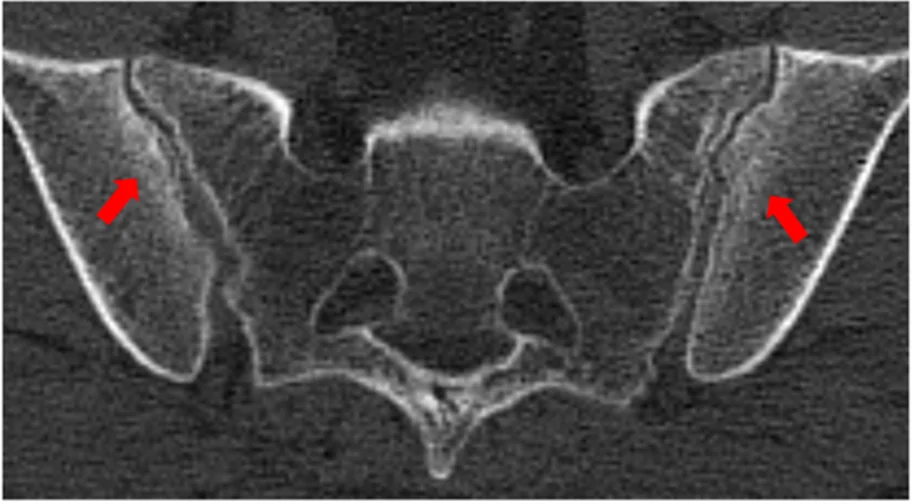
CT imaging demonstrated bilateral sacroiliitis. (arrows).
